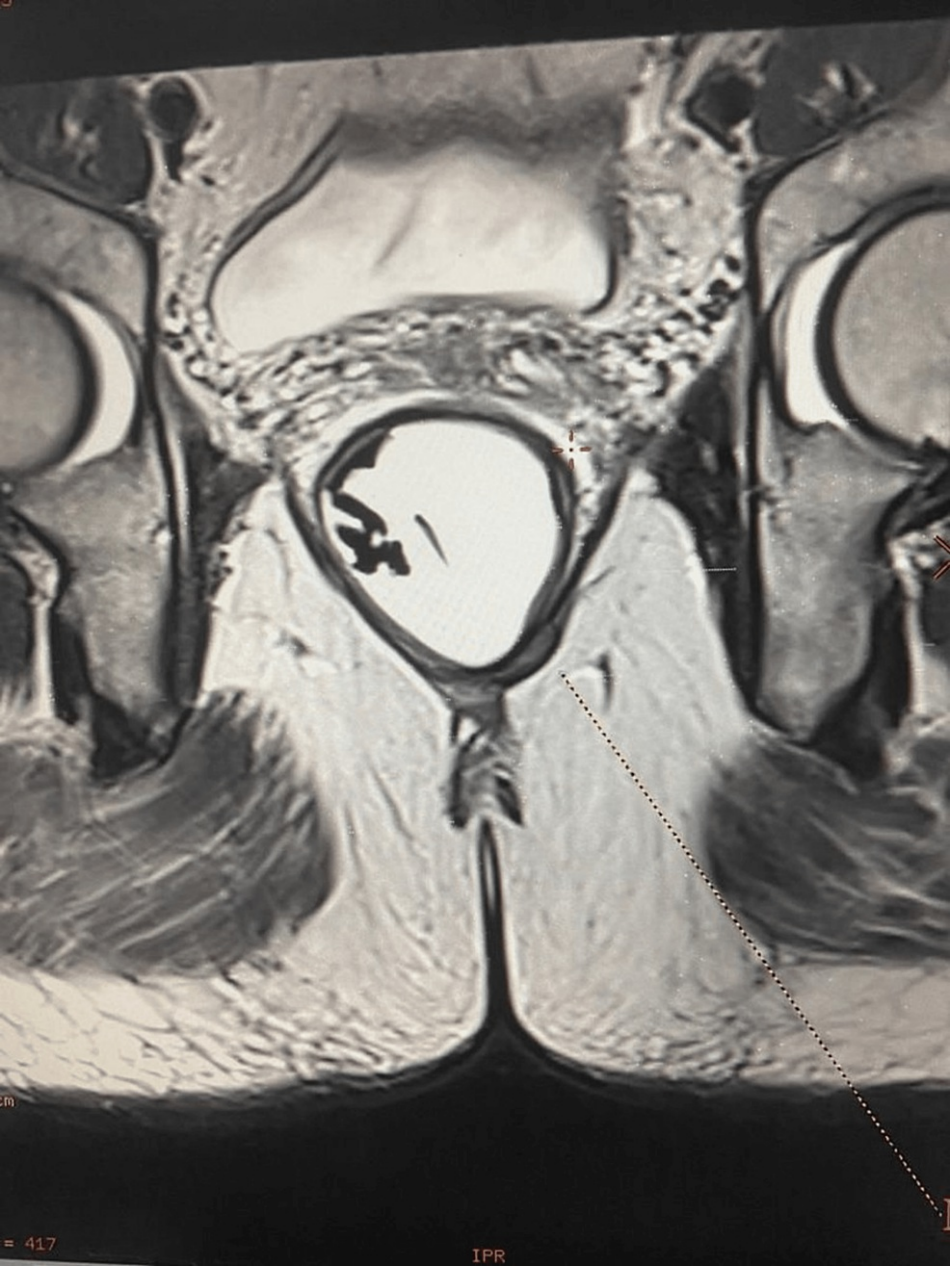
MRI finding of the residual lesion involving the left side of the anorectal junction adjacent to the intersphincteric space and the levator ani muscle.
